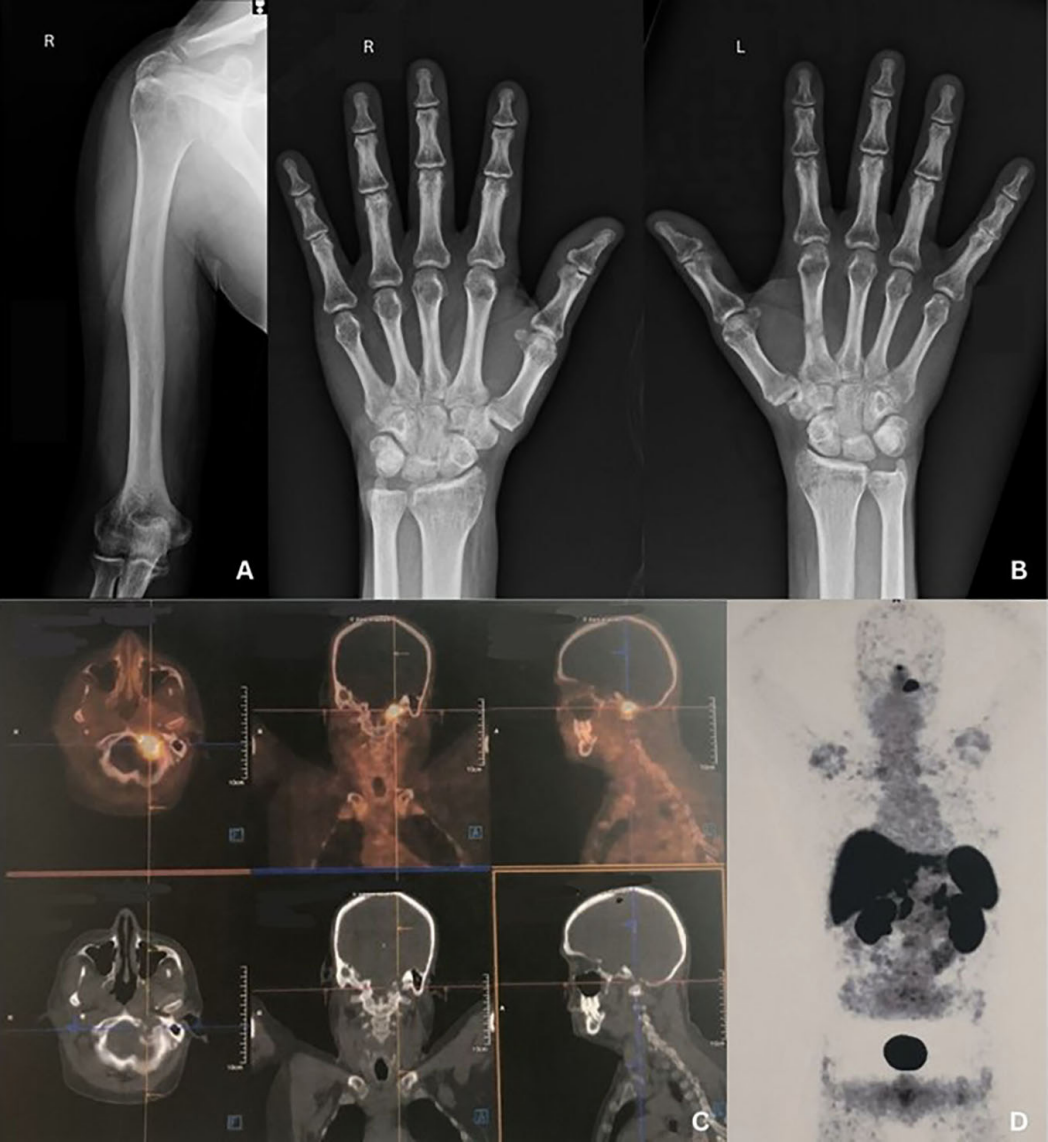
A and B, diffuse bone demineralization and bone deformities suggestive of osteomalacia. C, nodular ^68-^Ga-Dotatate-enhanced image of the left occipital condyle compatible with a tumor-inducing osteomalacia; D, PET-CT showing the location of the tumor and its representation of indirect metabolism.
